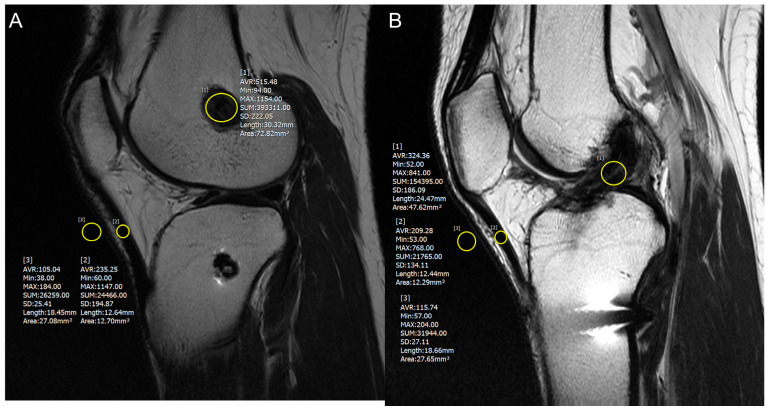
This is an 18-month follow-up MRI of a 42-year-old female patient after ACL reconstruction. Image (A) shows the measurement of the contrast-to-noise ratio (CNR) of the intra-tunnel ACL graft, while image (B) depicts the measurement of the CNR of the intra-articular graft. To measure the CNR of the intra-tunnel ACL graft, the region of interest (ROI) was drawn as large as possible at the most widened portion of the graft. For the CNR measurement of the intra-articular ACL graft, a circular ROI was centered on the middle third of the ACL graft, with its center aligned with the ACL’s anterior and posterior borders as its diameter. The ROI for the patellar tendon was drawn at its mid-portion, ensuring it was as large as possible while closely aligning with the anterior and posterior margins. Additionally, a background ROI was measured approximately 2 cm anterior to the patellar tendon on its anterior aspect.
